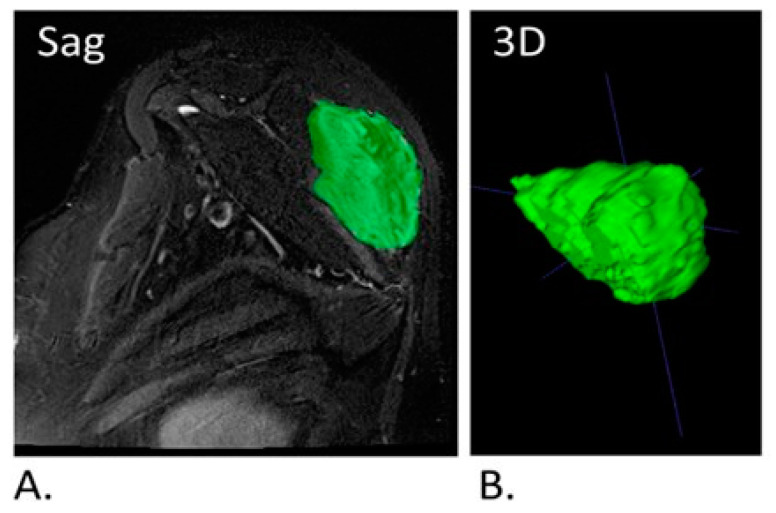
(A). Sagittal T2FS MRI of a segmented DT located in the soft tissues of the torso. (B). Extracted 3D model of the tumor seen in (A). DT; Desmoid tumor.
